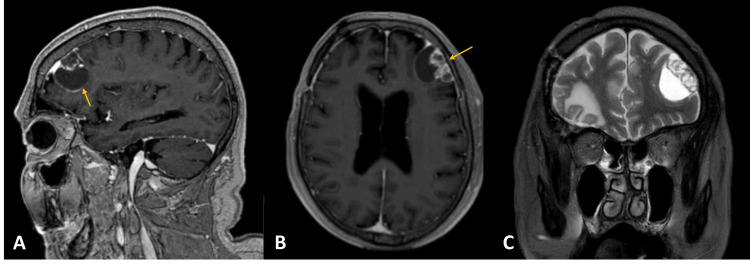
Cranial MRI showing ectopic recurrence Left frontal lobe extra-axial lesion with a solid and cystic component, with heterogeneous contrast enhancement. (A) Gadolinium-enhanced sagittal T1-weighted image; (B) gadolinium-enhanced axial T1-weighted image; (C) T2-weighted coronal image. Yellow arrow indicating the lesion.
